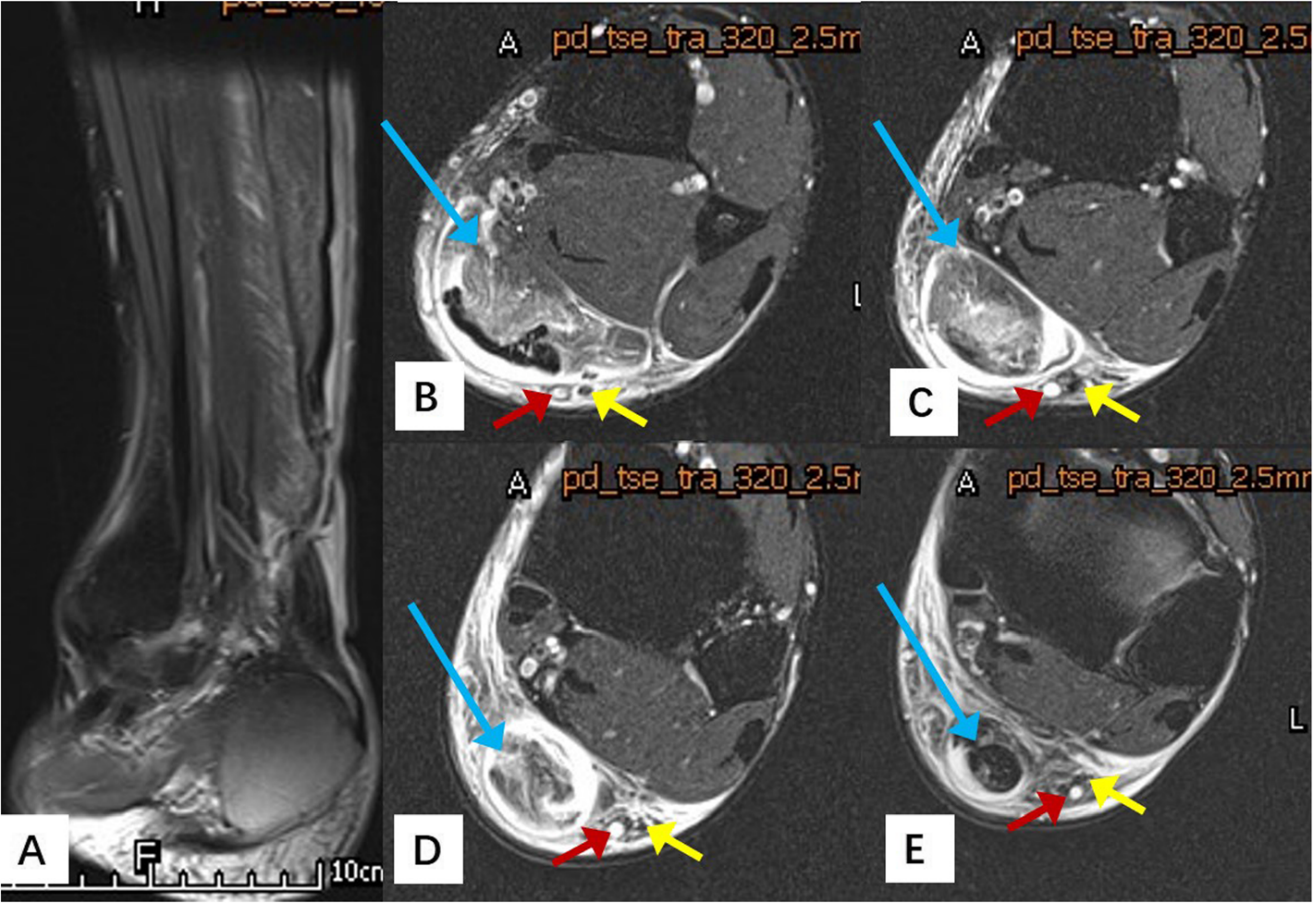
A male patient, 35 years old, with the Achilles tendon rupture in the basketball game. a. Magnetic resonance imaging scan shows an Achilles tendon rupture with retraction and gapping between the tendon ends on the sagittal image. b–e. Magnetic resonance imaging scan shows the relationship of the tendon, the sural nerve (SN), and the small saphenous vein (SSV) on the cross-sectional images. The blue arrow shows the ruptured tendon; the red arrow shows the small saphenous vein; the yellow arrow shows the sural nerve
